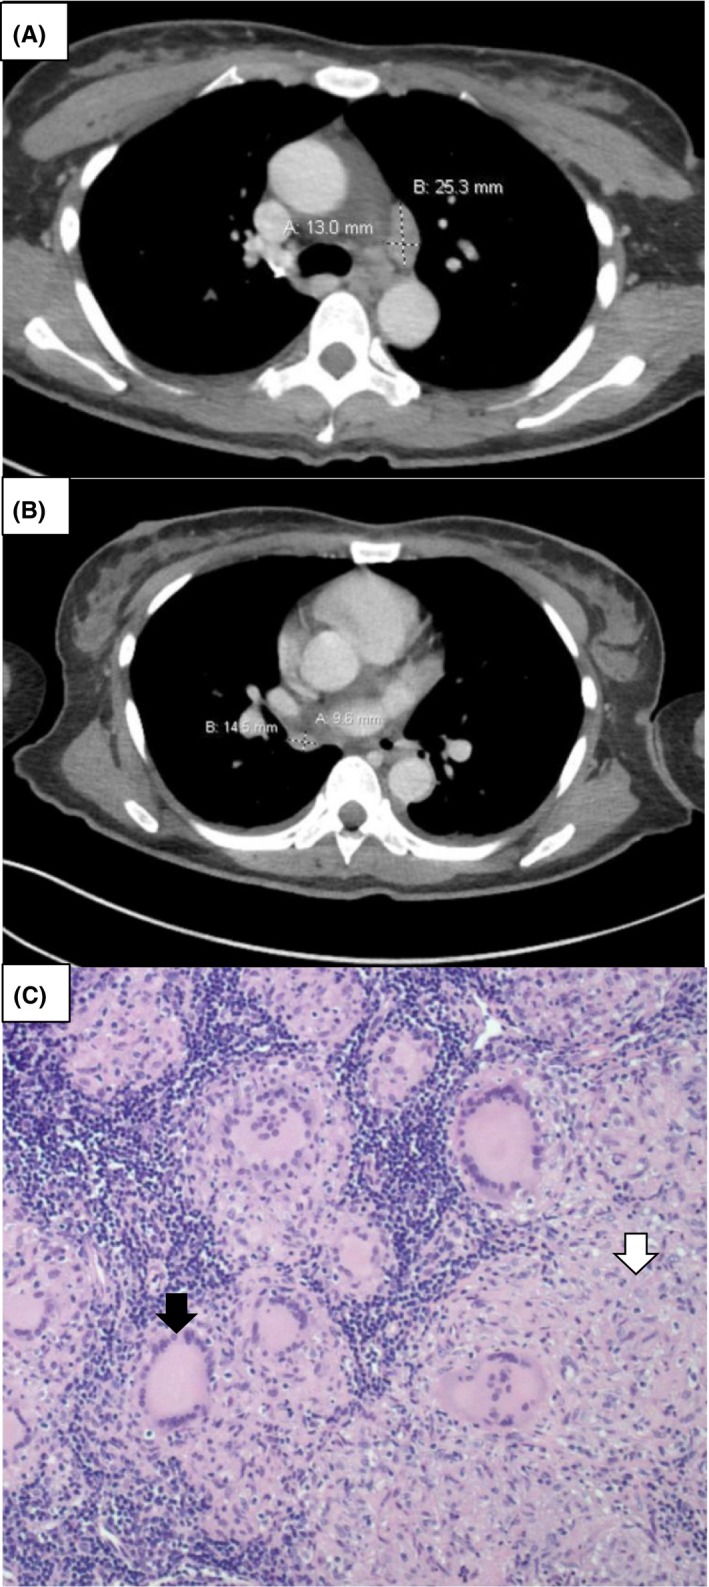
Chest CT demonstrating bilateral enlarged thoracic lymph nodes of 13.0 X 25.3 mm and 9.6 X 14.5 mm consistent with sarcoidosis (A, B). Lymph node biopsy demonstrating granulomatous inflammatory reaction with multinucleate giant cells (black arrowhead) and fibrosis (white arrowhead) (C)
